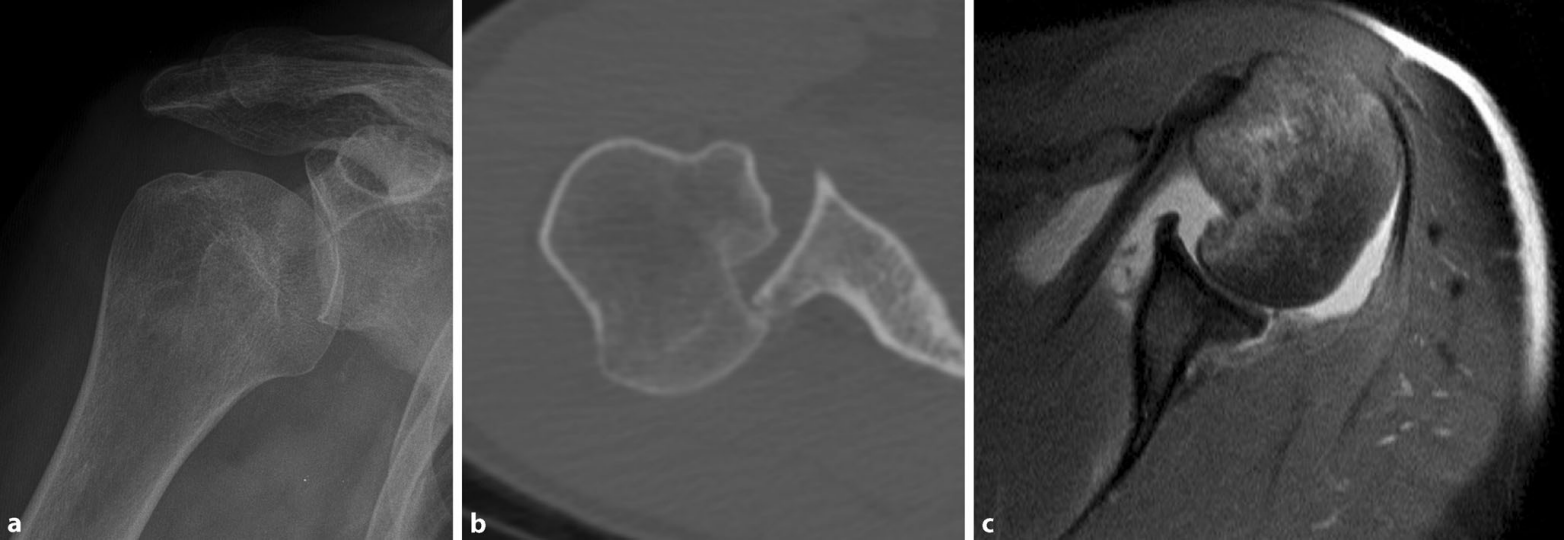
First-time posterior dislocation (A2) X‑ray (a) and computed tomography image (b) of an acute locked posterior shoulder dislocation with large reverse Hill–Sachs defect as well as magnetic resonance scan (c) of a reduced acute posterior shoulder dislocation with large Hill–Sachs defect and posterior Bankart lesion
